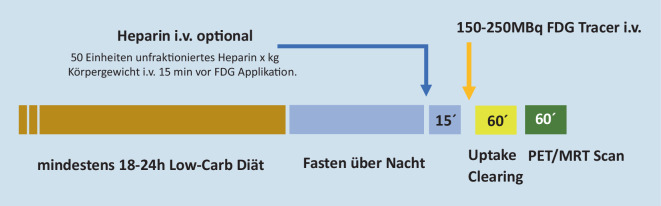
Unterstützt werden kann die myokardiale FDG-Suppression durch einen Heparinbolus (unfraktioniertes Heparin 50 IE/kg Körpergewicht) 15 min vor Tracerapplikation [10]. Allerdings kann Heparin eine mangelnde diätetische Vorbereitung nicht *retten* oder gar gänzlich ersetzen.
